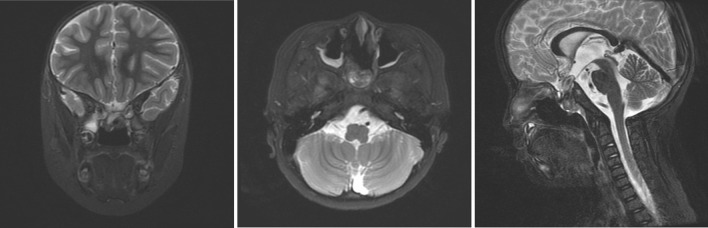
Postoperative MRI scan of the patient on 14th postoperative day. The MRI showed no cerebral edema, the encephalocele was largely reduced with only minor remnant tissue locating on the roof of the nasal cavity
